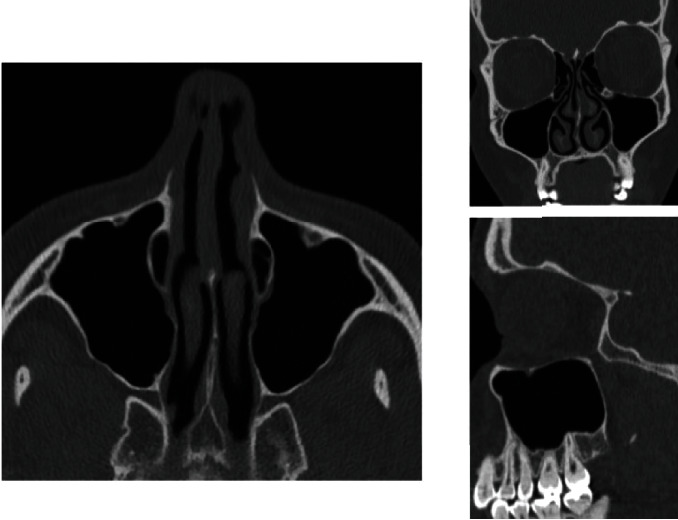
CT scan of the maxillary sinuses showing the anatomy of the sinuses and their relationship to the teeth.
